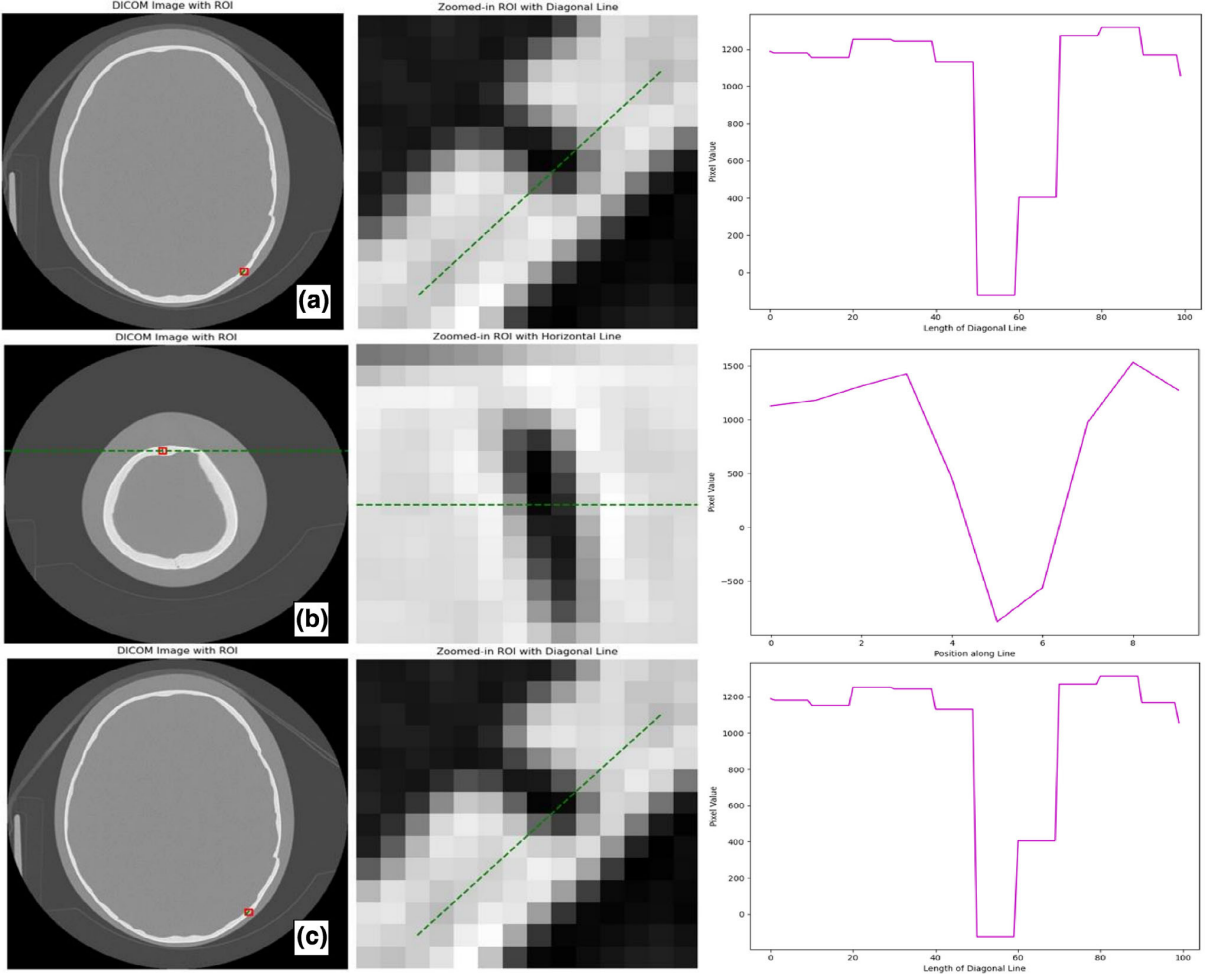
Line profile fitting for (a) Lambdoid, (b) Sagittal, and (c) coronal sutures, utilizing a standard pediatric CT protocol for the Canon Aquilion One. The acquisition parameters are detailed in Table 1, enabling the examination of pixel variations associated with the presence of each suture.
